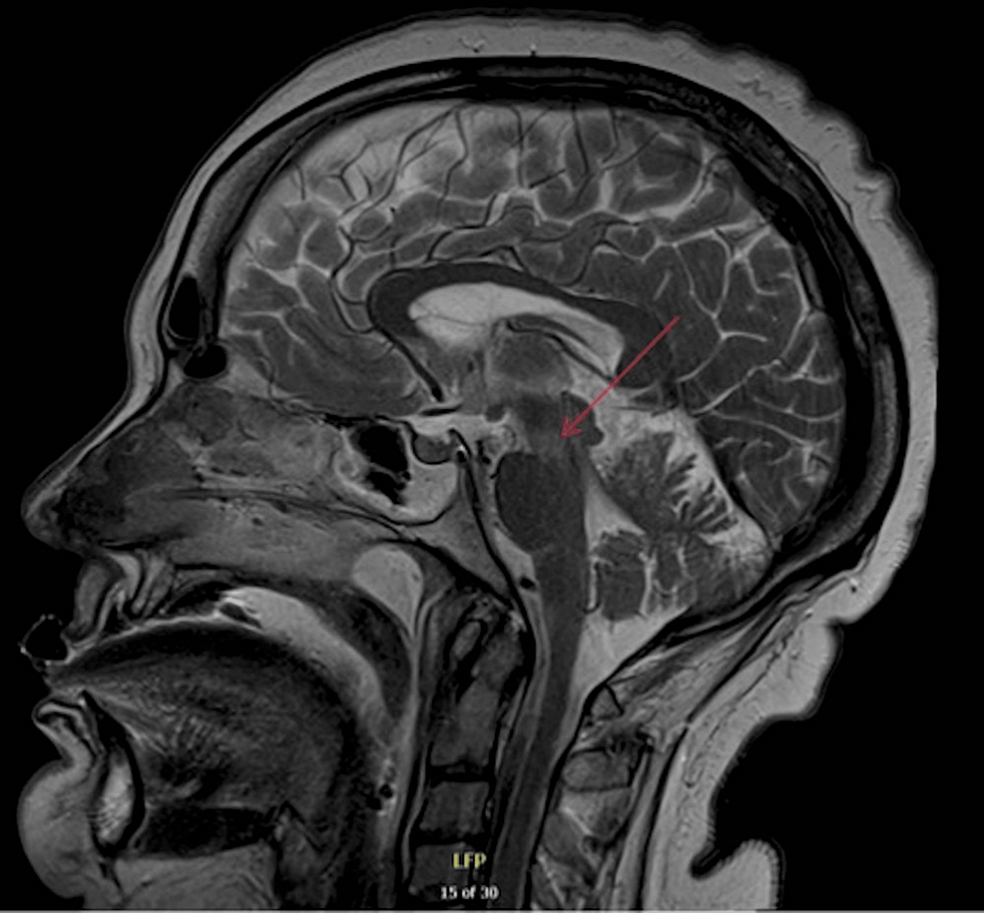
MRI head showing T2 hyperdensity at the mesocepahalopontine junction (red arrow) T2: T2 weighted image
